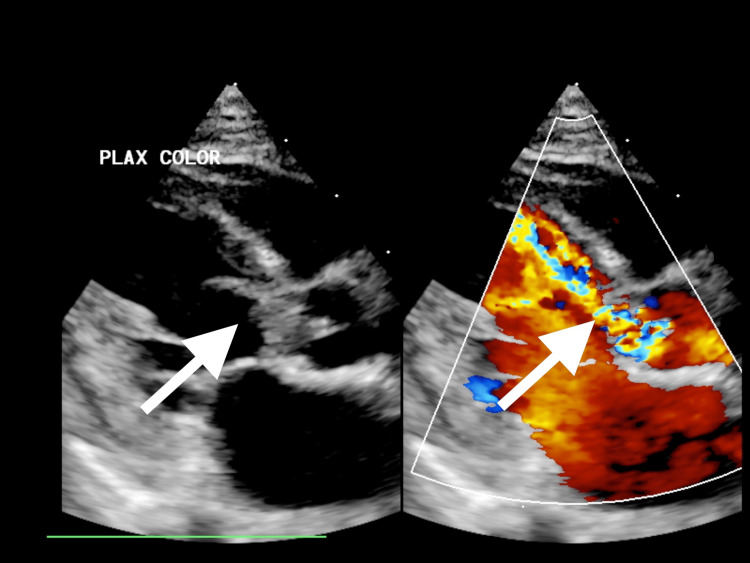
Long-axis parasternal view with color flow Doppler showing the same vegetation with significant aortic valve regurgitation due to destroyed aortic valve leaflets.
